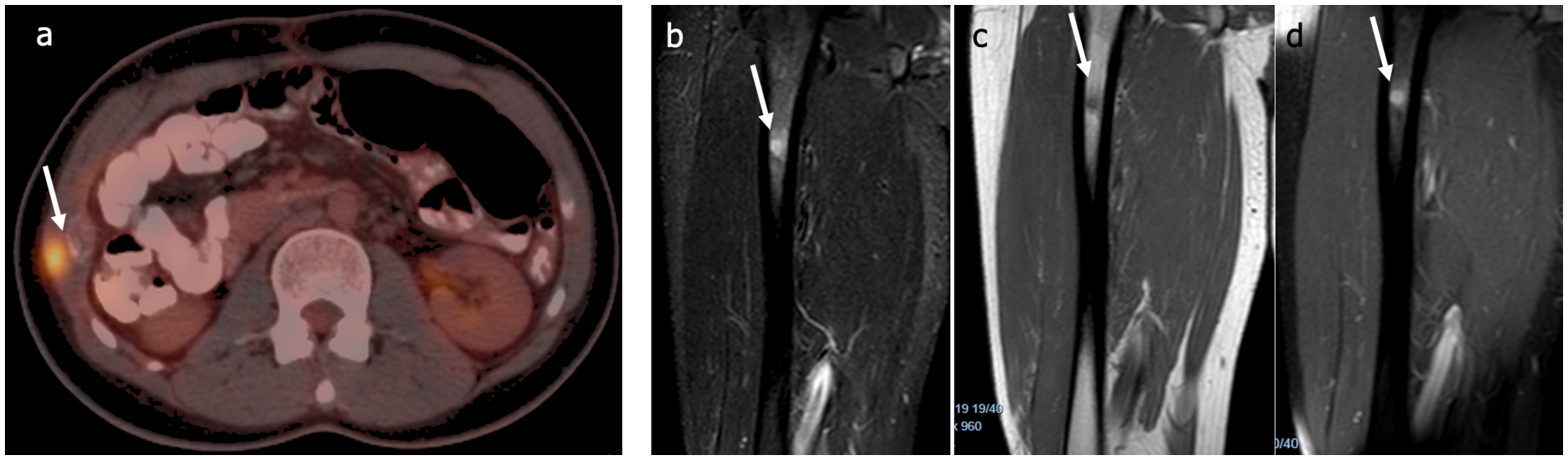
(a) Thoracic wall metastasis detected by PET-CT (arrow). The MRI of the femur showed an 8 mm intramedullary lesion (arrow), which was (b) hyperintense on T2 STIR sequences, (c) hypointense on T1-weighted sequences, and (d) enhanced contrast-enhanced T1-weighted sequences.
